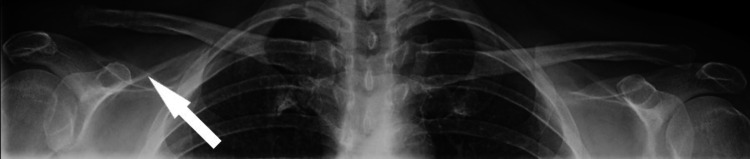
Bilateral anterior-posterior (AP) X-rays upon patient presentation with spontaneous recurrence of right acromioclavicular (AC) deformity and pain at eight weeks after hook plate removal
